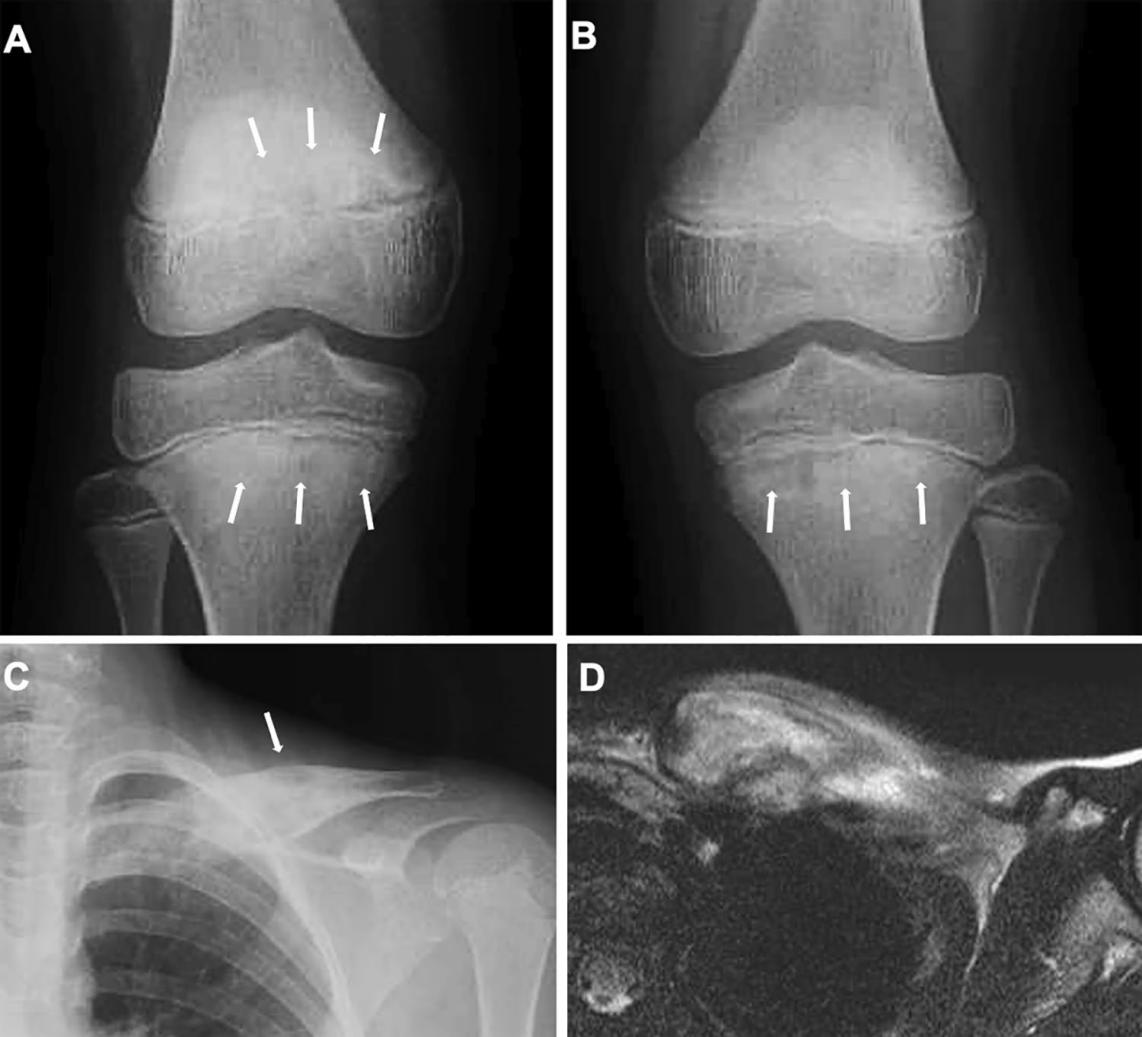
CRMO. A–D. A 9-year-old girl with recurrent left clavicular pain over several months and recent development of painful bilateral knees. The frontal radiographs of the knee demonstrate mixed sclerotic and lytic changes in the right distal femoral meteaphysis and bilateral proximal tibial metaphyses (arrows, A, B). The frontal radiograph of the left clavicle shows expansion and sclerosis of the medial and middle clavicular segments (arrow, C). Coronal fat-suppressed T2-weighted image reveals intraosseous high signal intensities of the thickened clavicle associated with surrounding soft tissue edema (D). There is no evidence of abscess formation
